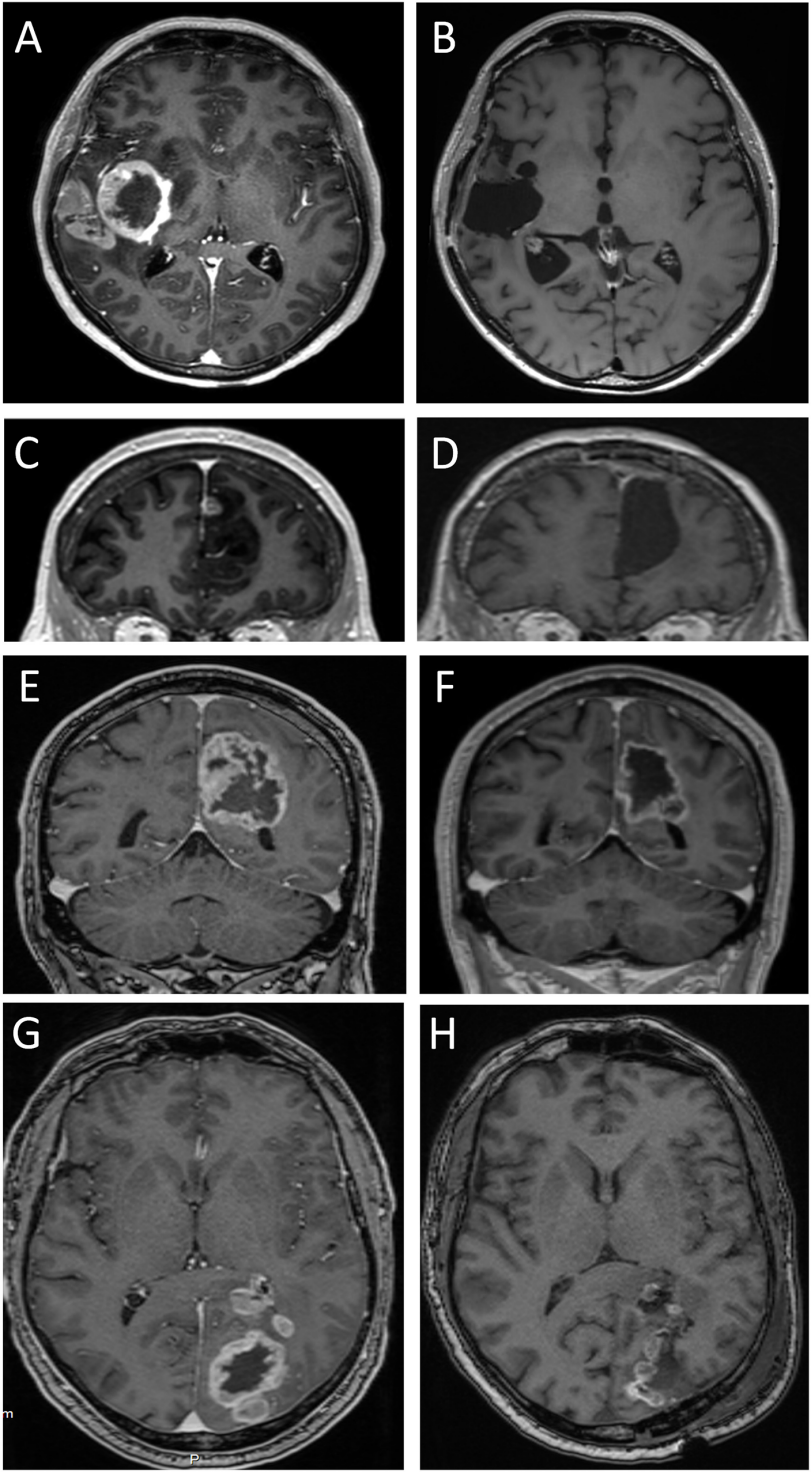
Four solid glioblastomas before and after tumor surgery. T1-weighted MPRAGE MRIs were obtained after intravenous injection of gadolinium-based contrast medium (which gives a bright, ring-formed signal in most images). Images (A, C, E, G) show pre-surgical MRIs. Tumors in images (A, C) were assessed as having minimal mass effect. Note the modest displacement of surrounding brain tissue (compare with contralateral side). Tumors in images (E, G) were assessed as having moderate and pronounced mass effect, respectively. Images (B, D, F, H) show MRIs after 400, 167, 26, and 4 days, respectively. Note the absence of brain tissue where the tumor resided. This includes both white matter and the overlying neocortex.
